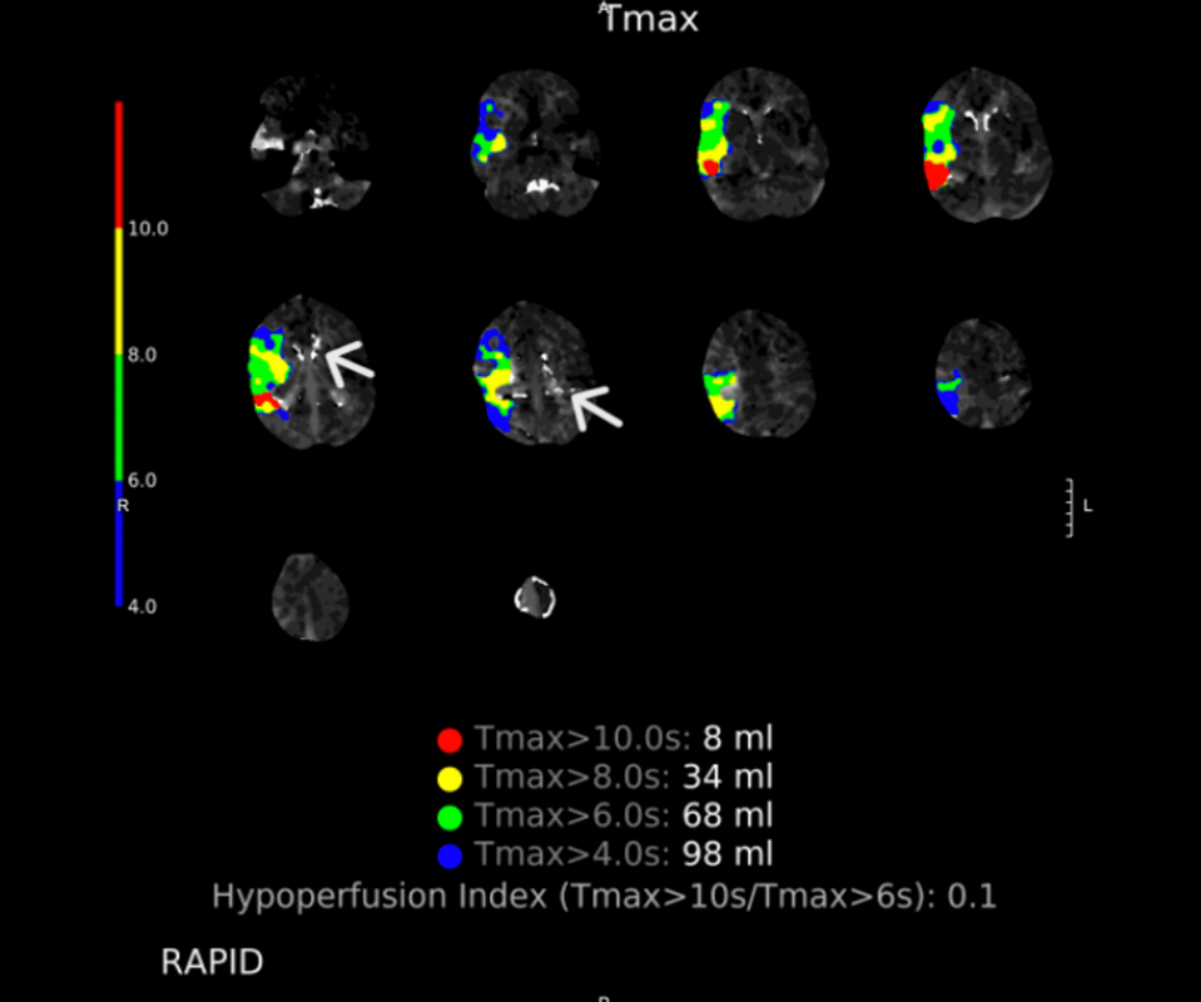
Computed tomography angiogram demonstrating perfusion defect at the right M1 segment of the right middle cerebral artery. White arrows represent calcifications in the thalamus and basal ganglia.
